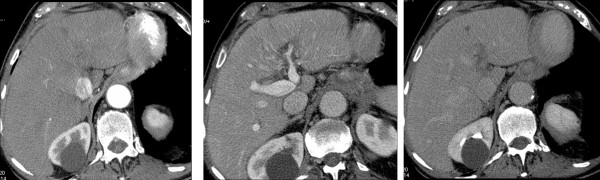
three-months follow-up with CT: 2a, arterious phase; 2b, portal phase; 2c, late phase. The three months CT scan document an apparent reduction in the size of lesion, which is associated with partial recanalization of the left portal branch.
